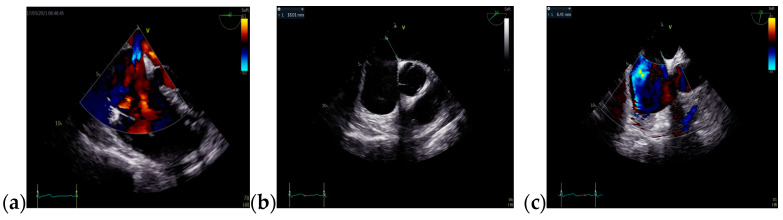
TOE: (a) Mid-esophageal 4-chamber view: turbulent color Doppler flow at the level of the interatrial septum; (b) Mid-esophageal short-axis view: atrial septal defect with a diameter of 1.8 cm (18 mm); (c) Mid-esophageal short-axis view: aortic rim = 6.4 mm. Abbreviations: TOE—Transesophageal echocardiography.
